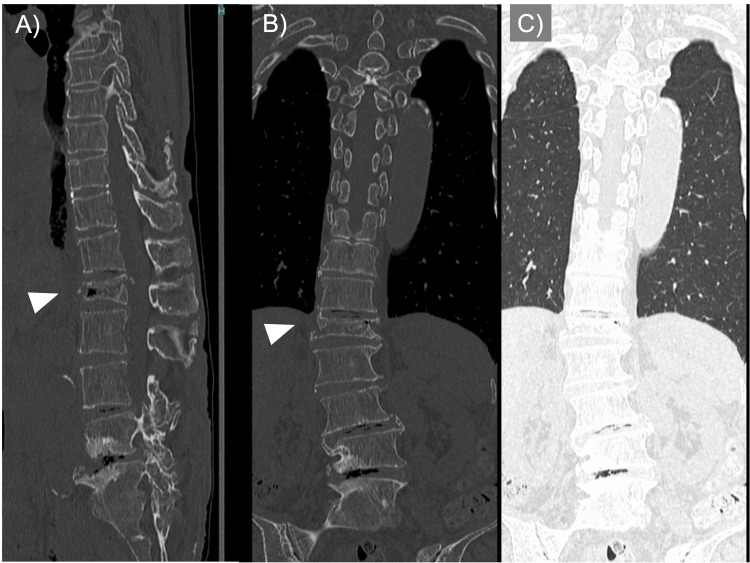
Preoperative CT Images of the Lumbar Spine and Thorax Preoperative sagittal (A), coronal (B), and coronal lung-window (C) CT images confirming the absence of pneumothorax. The arrowheads in A and B indicate the fractured vertebra.
